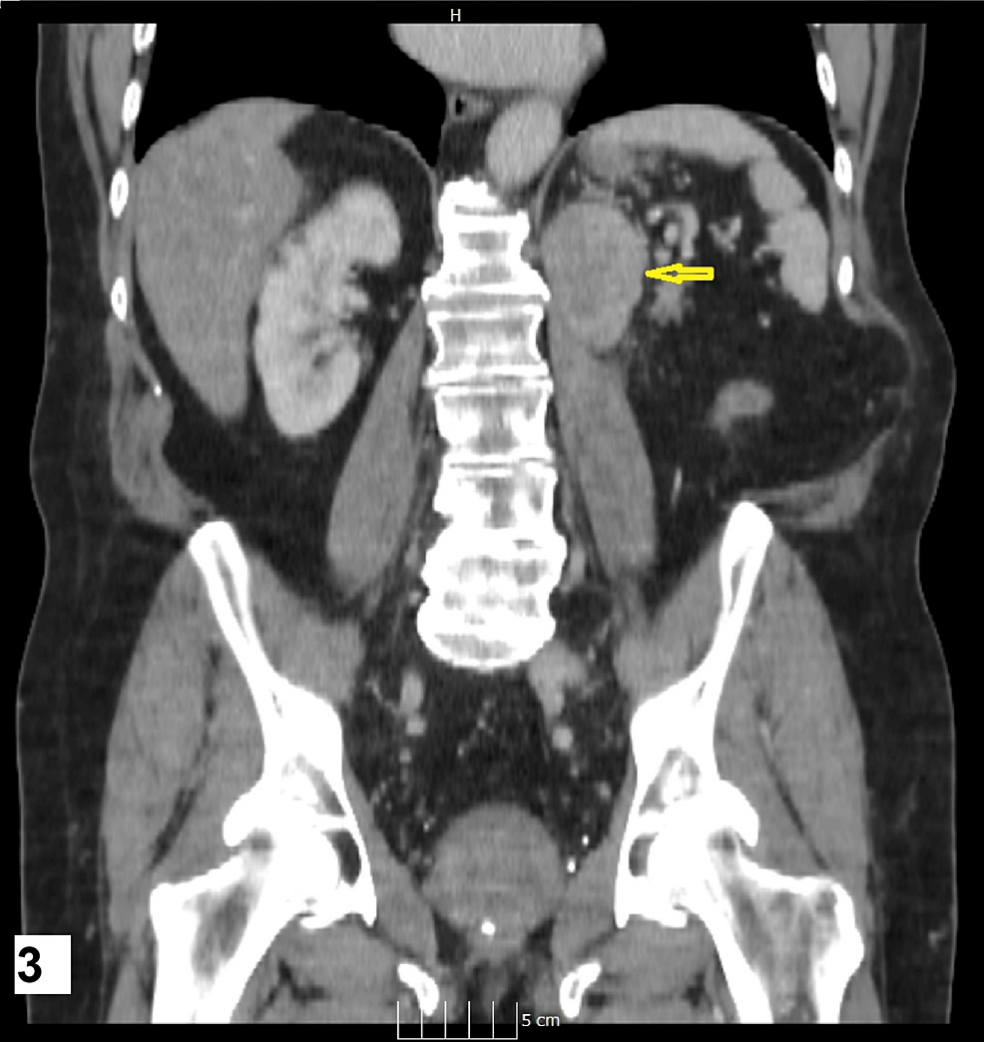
Coronal image of the CT scan showing the tumour recurrence site The yellow arrow points to the left nephrectomy bed recurrence.
